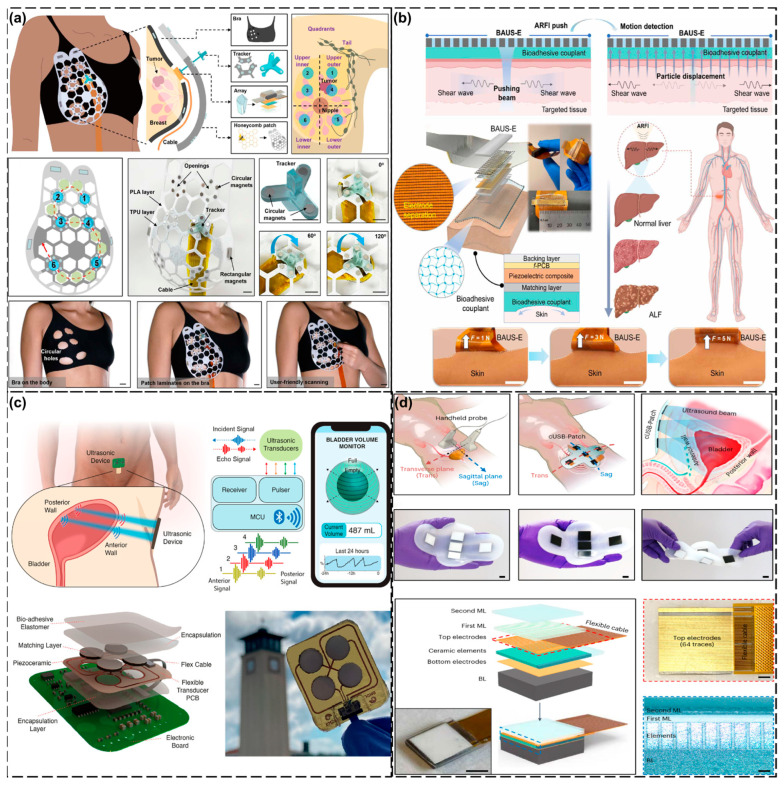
Wearable ultrasound testing in the chest and abdomen. (a) Ultrasound breast patch used for deep tissue scanning and imaging. Reproduced with permission: Copyright 2023, Science Advances [124]. (b) Design and mechanism of wearable BAUS-E. Reproduced with permission: Copyright 2024, Science Advance [125]. (c) Design and working principle of integrated ultrasonic device. Reproduced with permission: Copyright 2024, Springer Nature [126]. (d) Overview of phased array and cUSB-Patch. Reproduced with permission: Copyright 2023, Springer Nature [127].
